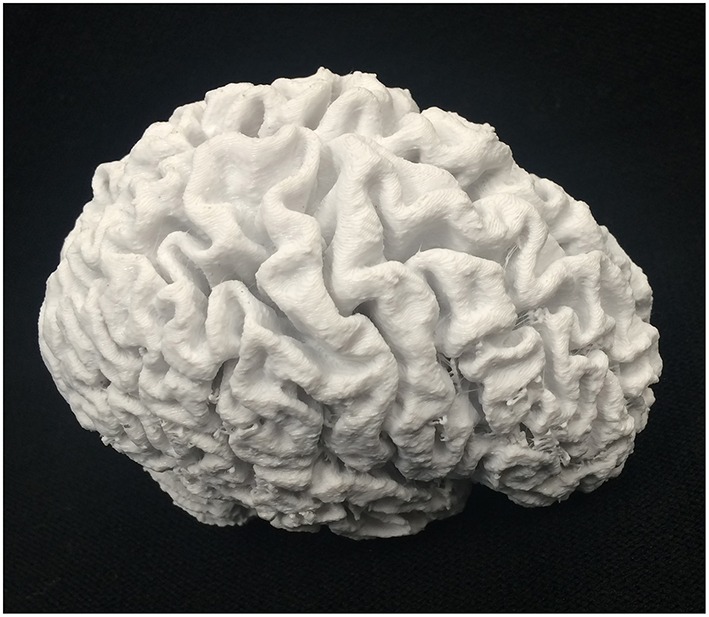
Brain 3D model, printed from an MRI image on the MakerBot Replicator 2 3D printer.
